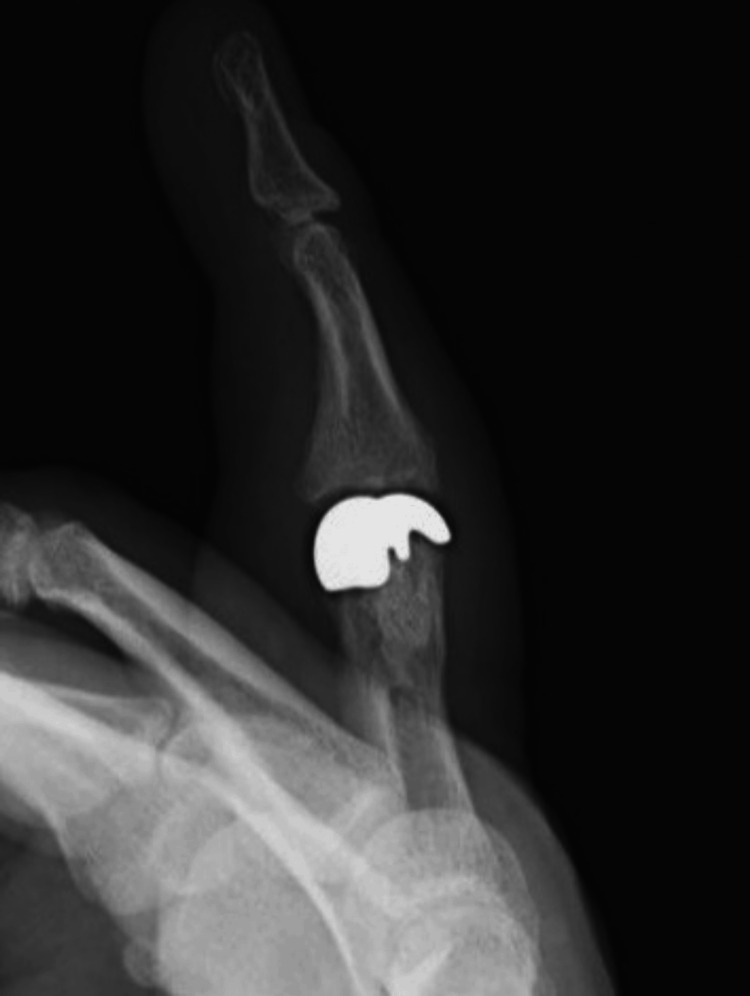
PIP-aimed X-ray obtained eight months after surgery PIP: proximal interphalangeal
